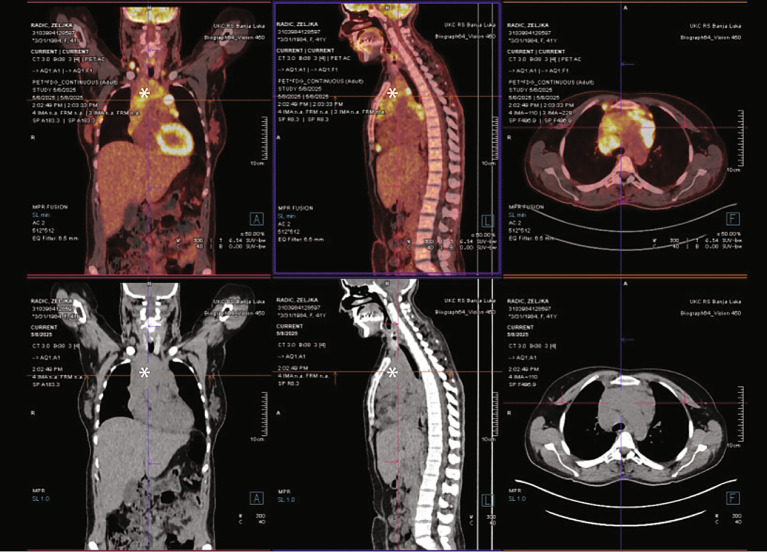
Comparison of contrast-enhanced CT and PET/CT images of the mediastinal mass (*). The CT images show bulky mediastinal lymphadenopathy, while the PET/CT images demonstrate intense FDG uptake in the same regions, highlighting metabolically active disease.
